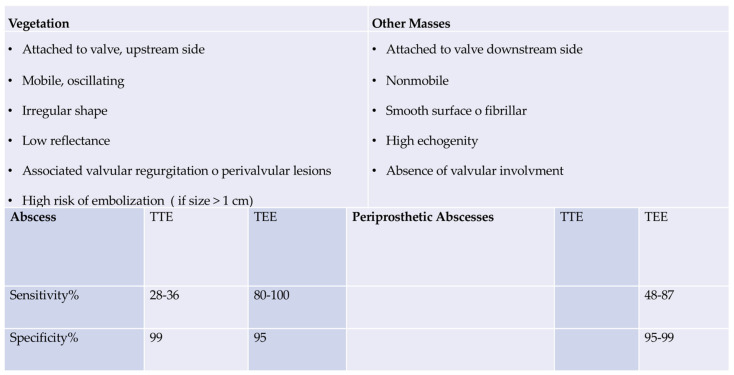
Sensitivity and Specificity of Echocardiography in detecting abscesses. Fowler, V.G. et al *Clin. Infect. Dis.* 2023, *77*, 518–526. Ref [5].
